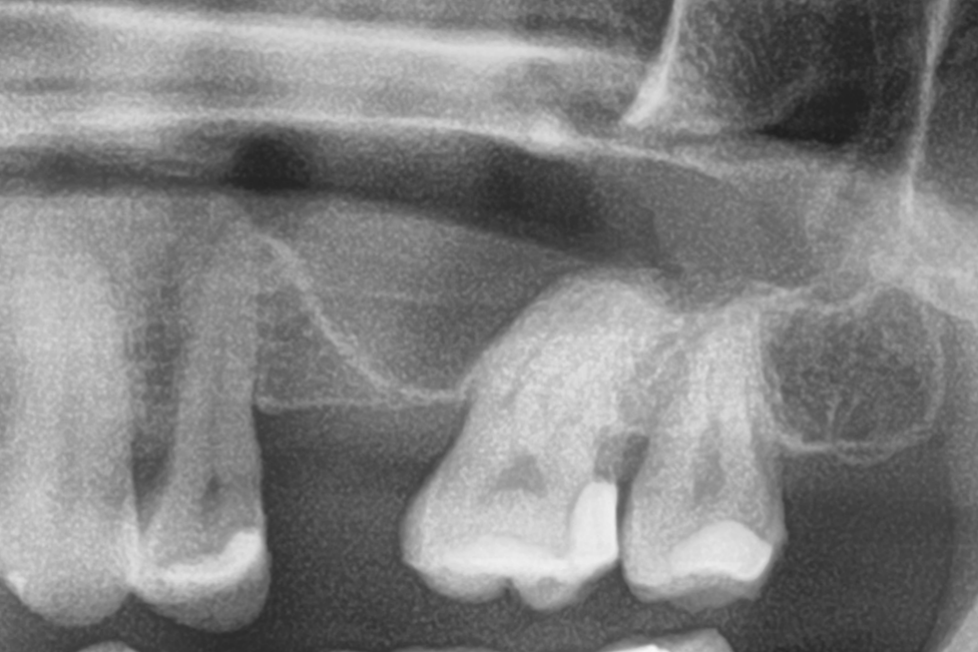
Preoperative radiograph with a planned implant in regio 26
